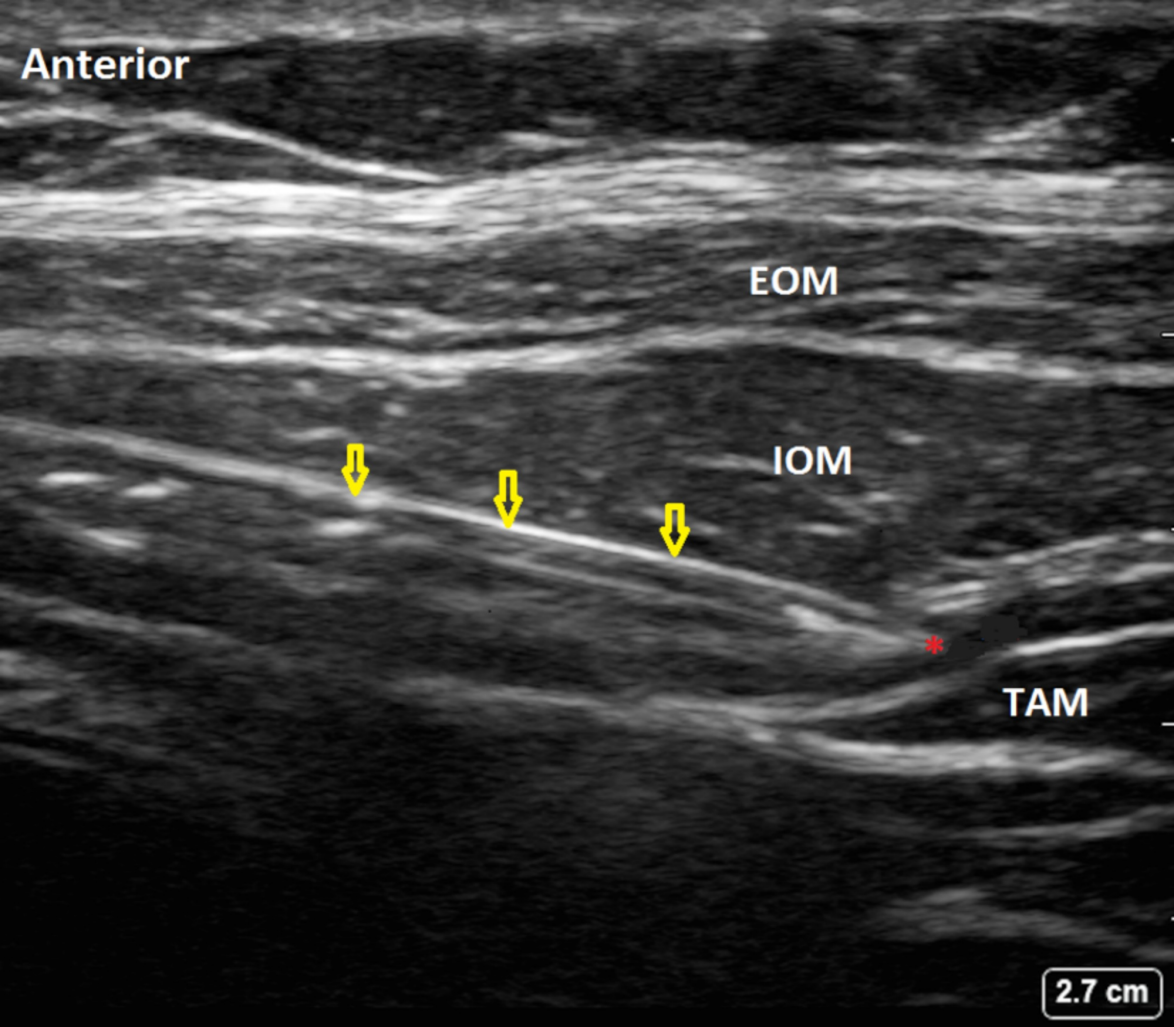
Ultrasound-guided Transversus Abdominis Plane (TAP) Injection. The yellow arrows indicate the needle, and the red (*) indicates the injection site. EOM: External oblique muscle; IOM: Internal oblique muscle; TAM: Transversus abdominis muscle.
